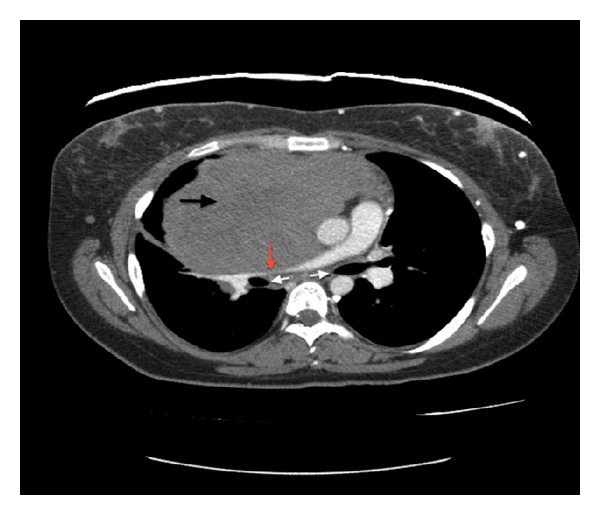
CT scan of the chest with contrast reveals a large lobulated anterior mediastinal solid mass (black arrow) with extension into the right hemithorax and the right atrium. There is displacement of the great vessels into the left hemithorax with significant mass effect on the right upper lobe. The tumor causes compression of the right pulmonary artery (red arrow) and right and left mainstem bronchi (white arrows).
